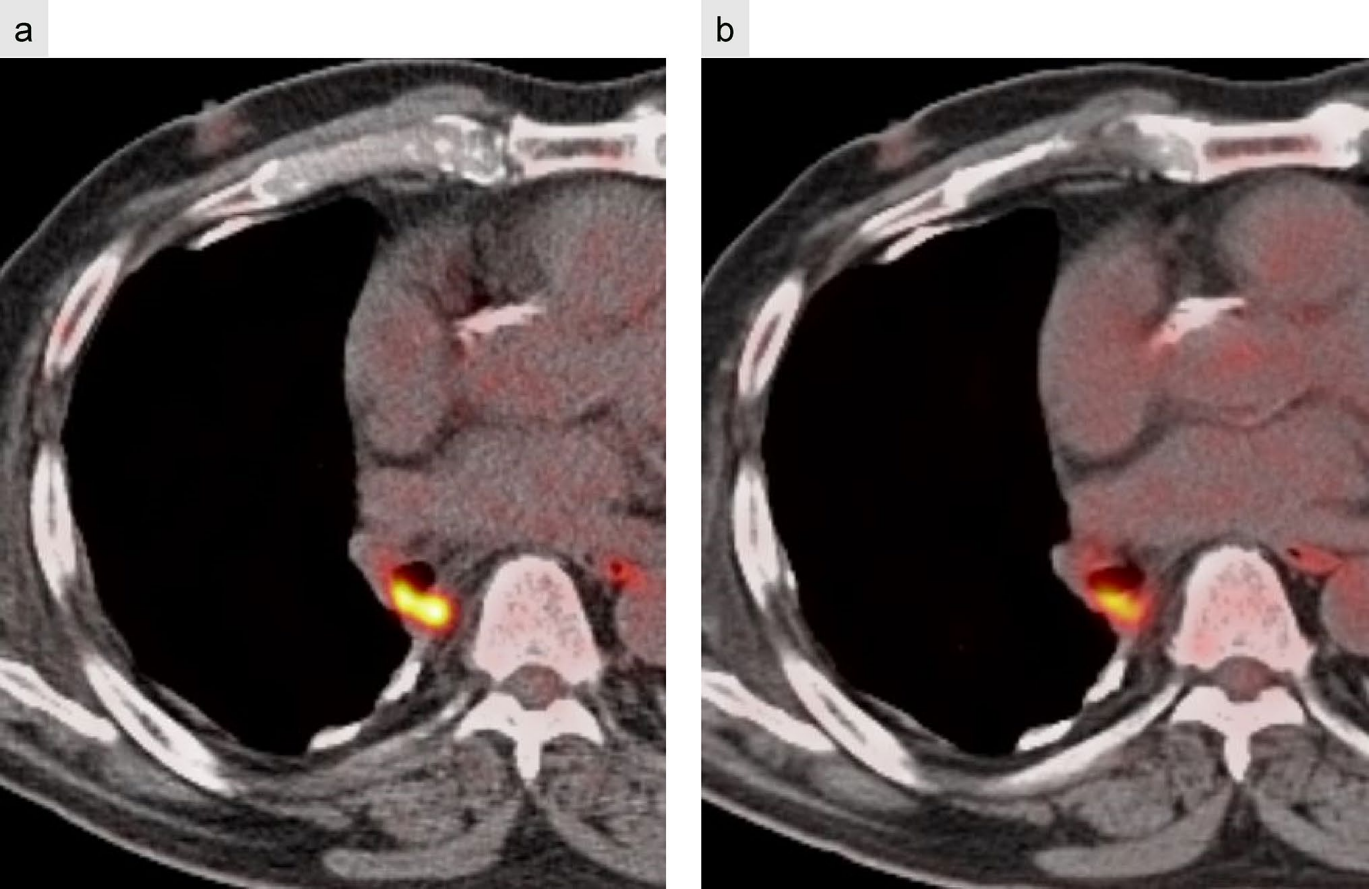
Staple line granuloma. FDG-PET/CT performed 3 years postoperatively shows increased FDG uptake at the bronchial stump (a). Bronchoscopy confirms no evidence of tumor recurrence. At the 5-year follow-up (b), FDG uptake persists but is slightly reduced, consistent with granulation tissue
